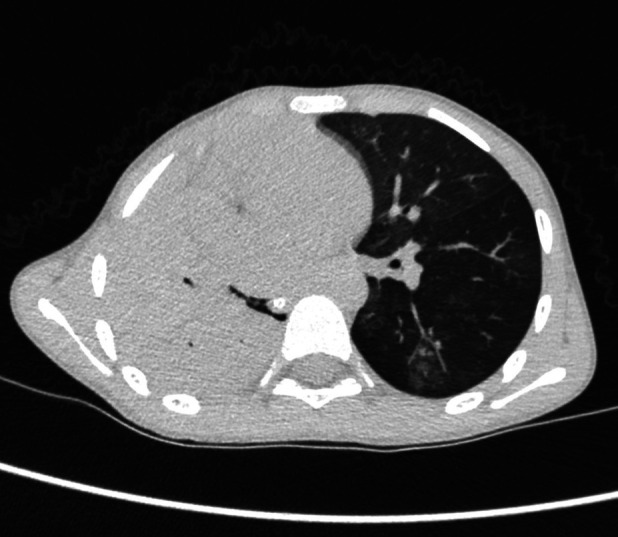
The initial CT scan at admission for case 3.
